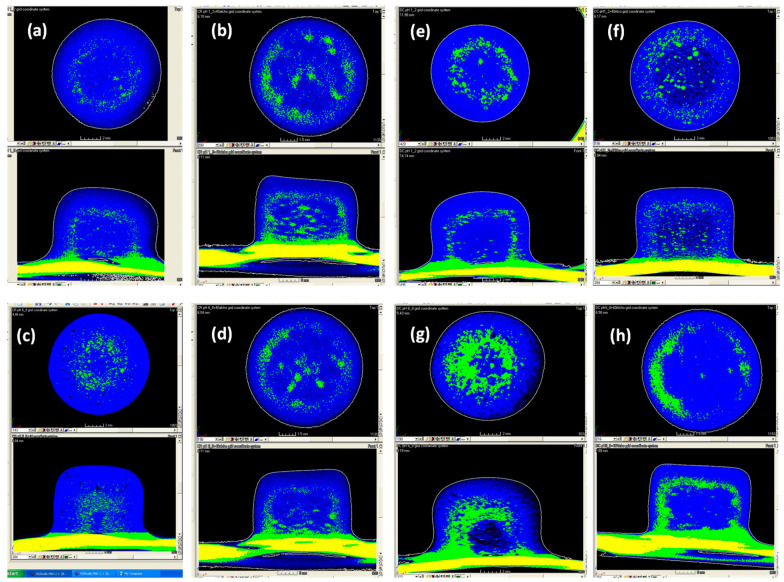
X-ray micro-tomographic images of the sagittal and transverse sections of the HPMC CR formulations in (a) pH 1.2 (0% absolute ethanol), (b) pH 1.2 (40% absolute ethanol), (c) pH 6.8 (0% absolute ethanol) and (d) pH 6.8 (40% absolute ethanol) for up to 7 h. X-ray micro-tomographic images of the sagittal and transverse sections of the HPMC K100M DC formulations in (e) pH 1.2 (0% absolute ethanol), (f) pH 1.2 (40% absolute ethanol), (g) pH 6.8 (0% absolute ethanol) and (h) pH 6.8 (40% absolute ethanol) for up to 7 h.
